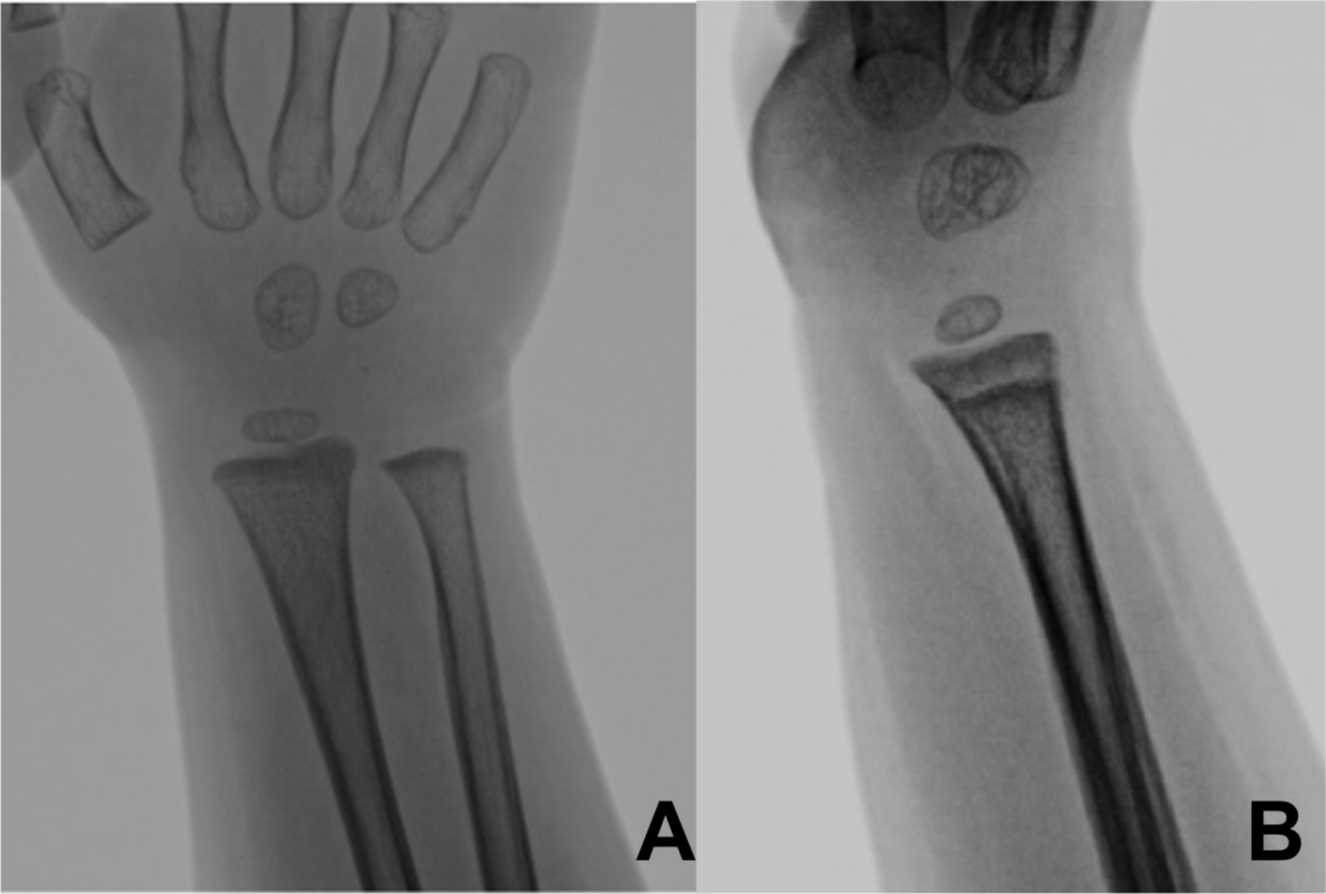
Anteroposterior (A) and lateral (B) radiographs of the wrist two years postoperatively with no evidence of bony abnormalities or growth arrest
